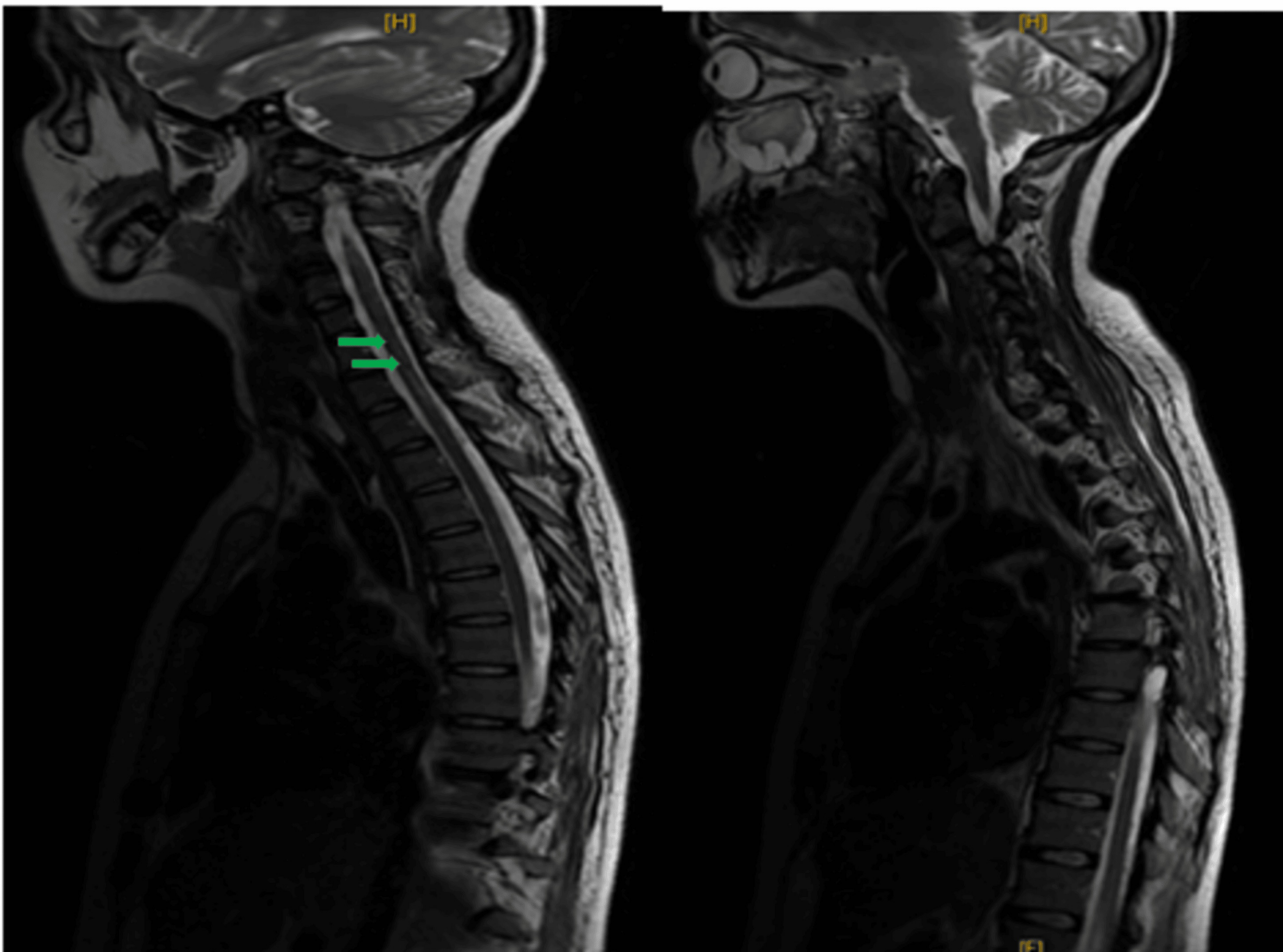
Follow-up sagittal T2-weighted MRI after immunotherapy Follow-up sagittal T2-weighted image showing partial regression of the cervical intramedullary hyperintense lesion after treatment (arrow). MRI: Magnetic resonance imaging
